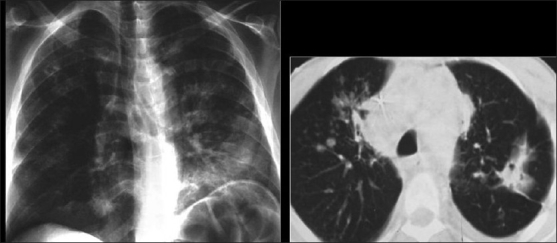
Chest X-ray and computed tomography showing features of reactivation *Mycobacterium tuberculosis* as patchy consolidation, including involvement at unusual sites, e.g. lower lobes as seen here, cavitation, nodularity and adenopathy
